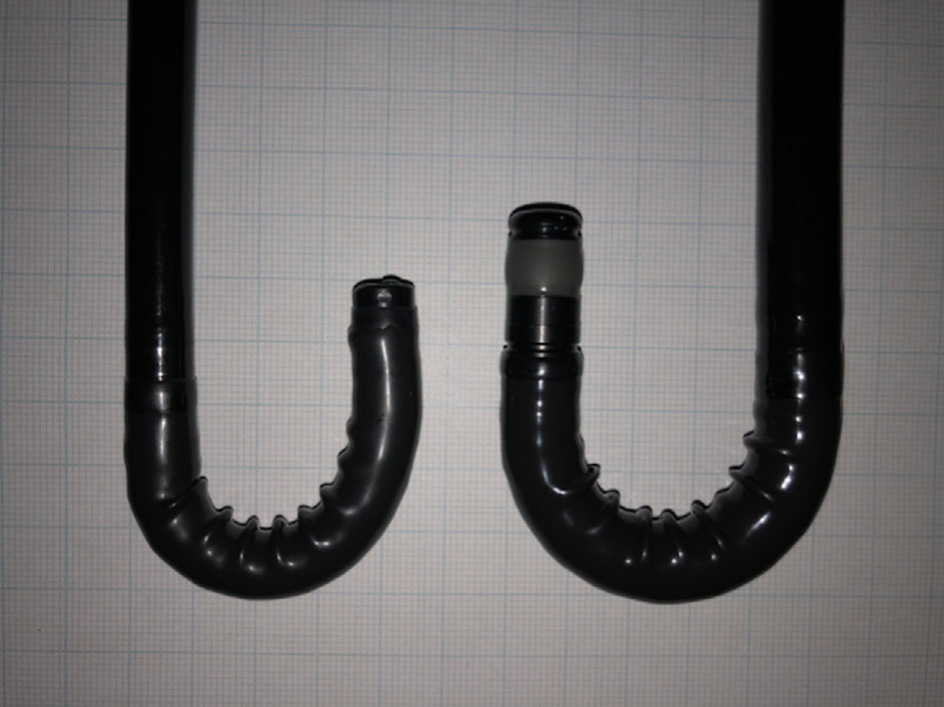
Photographs of standard EGD and forward‐viewing radial EUS bent at 180° upward. Left, standard upper EGD (EG‐L 600 WR7; Fujifilm, Tokyo, Japan). Right, forward‐viewing radial EUS (EG‐580UR; Fujifilm). EGD, esophagogastroduodenoscopy; EUS, endoscopic ultrasonography
